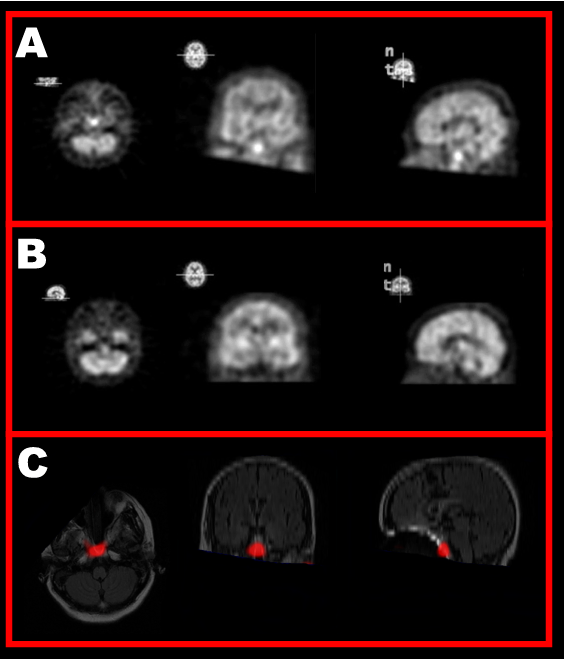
A: Axial, coronal and sagittal 99mTc-HMPAO SPECT images of the brain obtained during a facial twitching episode show moderate to severe heterogeneous cerebral hypoperfusion with an apparent focus of increased radiotracer uptake near the midbrain. B: 99mTc-ECD SPECT image of the brain obtained two weeks after (A) during a period free from twitching shows resolution of the area of increased uptake. C: Computer-generated depiction of A-B overlayed onto a co-registered MRI shows the area of increased uptake to lie outside the brain. See [5] or  for image analysis and alignment methodology.
